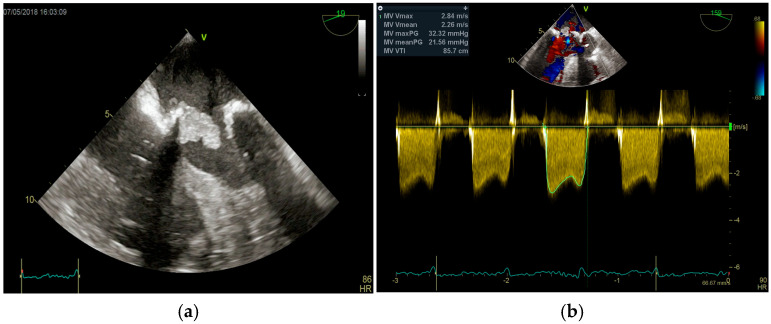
(a,b): Huge mitral valve endocarditis (a) determining fixed anterograde flow obstruction (mitral stenosis with Doppler tracing shown on the right). This is an original, de-identified clinical image from our institution. Patient consent for publication was obtained.
